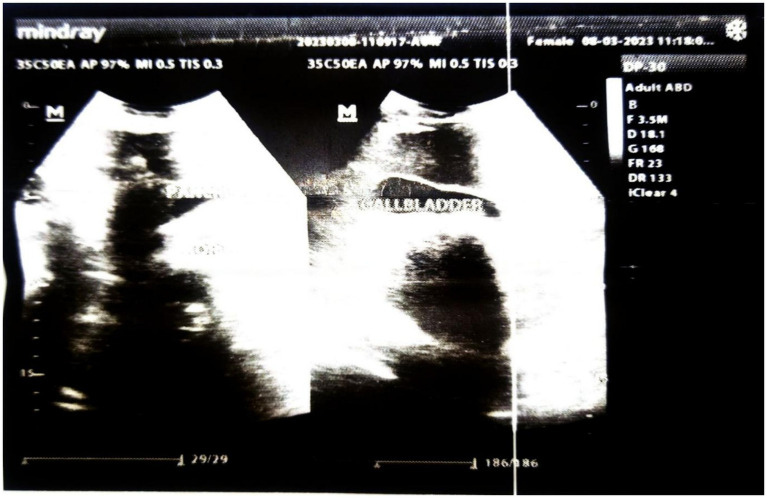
An ultrasound scan showing a normal pancreas and gallbladder (August 2023).
